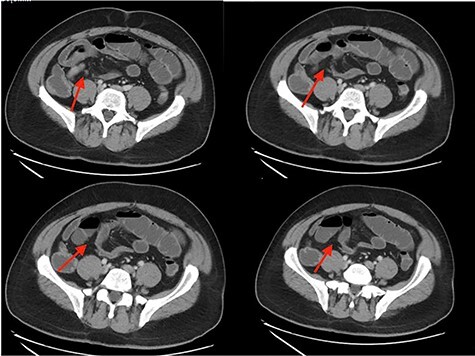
CT abdomen/pelvis showing SBO with transition point in right lower quadrant.
